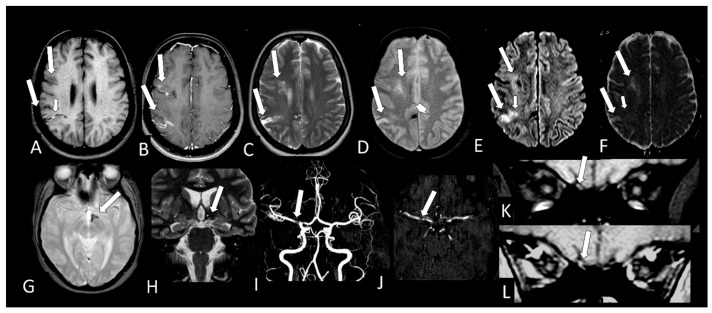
Case 34—The MRI shows a predominantly chronic ischemic stroke, located in the cortical and subcortical region of the right middle cerebral artery territory (M8 and M10) (A–F, long arrows). In the right M10 territory, the lesion has a small hyperintense “line” on T1 (A, short arrow) probably methemoglobin; the cortical enhances with contrast (B, long arrows); is hyperintense on T2 and T2*(C,D, long, arrow), being hyperintense in DWI (E, long arrows) and hyperintense on ADC-Map (F, long arrows), representing facilitates diffusion (T2 shine through effect). However, there is still a small area in M10 lesions that shows restricted diffusion of probably more recent ischemic injury being hyperintense in DWI (E, small arrow) and hypointense in ADC-Map (F, small arrow). There are small areas of bleeding with magnetic susceptibility in T2 * (D, arrow head) located in the medial cortical of the right cerebral hemisphere and in the left wall of hypothalamus (G, arrow) which is reduced in volume on coronal T2WI (H, arrow). On the angio MRI, parietal irregularity and narrowing in the right M1 is observed (I,J, arrow) and also in the origin of right anterior artery. Small dot of hyperintensity in the right olfactory bulb (K, arrow) observed on pre contrast fat suppressed T1WI, which seems have increased signal with contrast (L, arrow) representing probably methemoglobin and enhancement.
